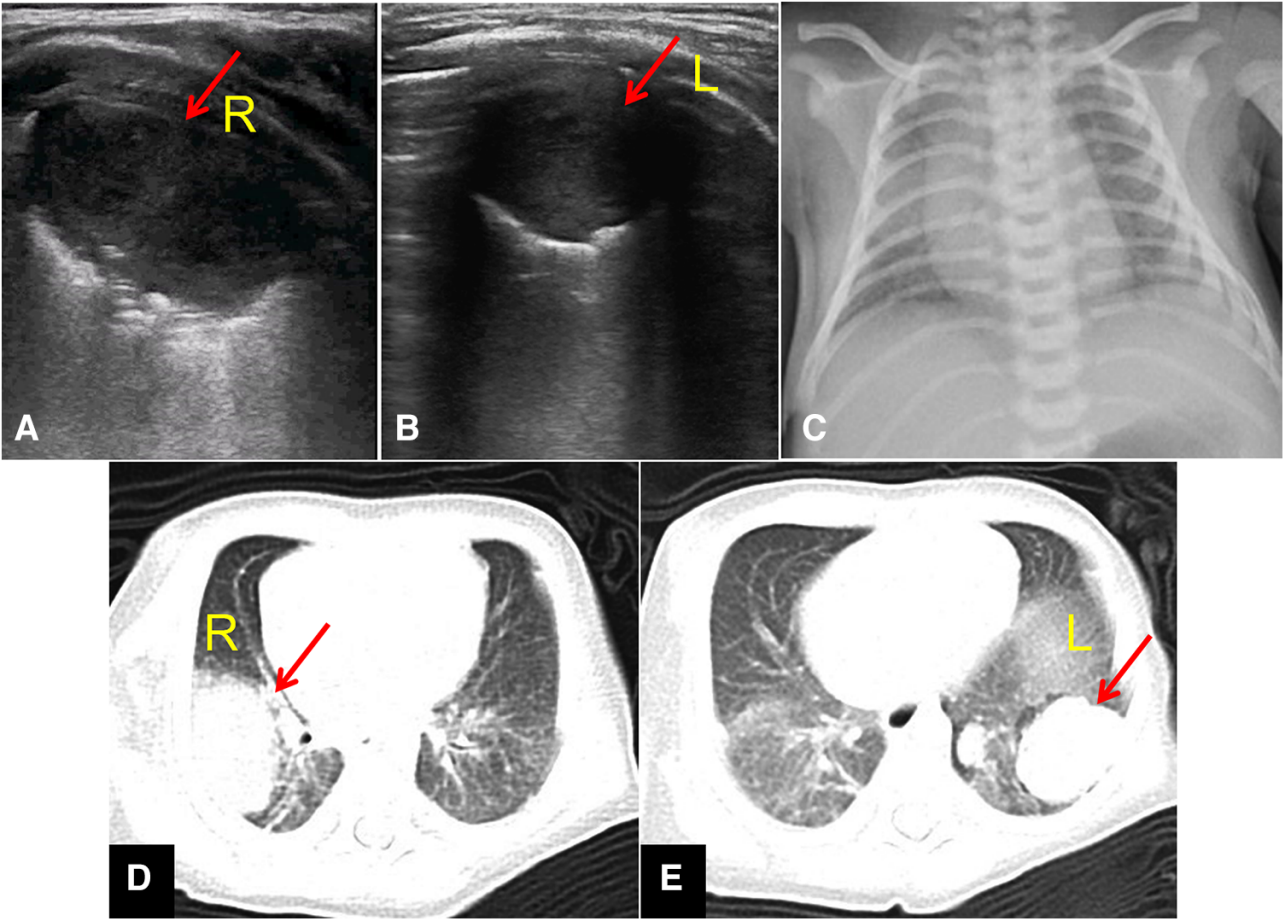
Imaging examination upon admission. (A) Lung ultrasound: elliptical consolidation lesions in the right axillary area (Red arrow, 32.3 mm^3^ × 20.2 mm^3^ × 18.1 mm^3^). (B) Lung ultrasound: elliptical consolidation lesions in the left lower lung (Red arrow, 22.2 mm^3^ × 16.4 mm^3^ × 15.8 mm^3^). (C) Chest x-ray examination: indicating right pneumonia. (D) An elliptical soft tissue mass shadow in the posterior segment of the right upper lobe (Red arrow, 31 mm^3^ × 22 mm^3^ × 20 mm^3^), with a large necrotic area in the center. (E) Multiple nodules and lumpy shadows in the lower lobe of the left lung (Red arrow, up to approximately 25 mm^3^ × 22 mm^3^ × 15 mm^3^).
